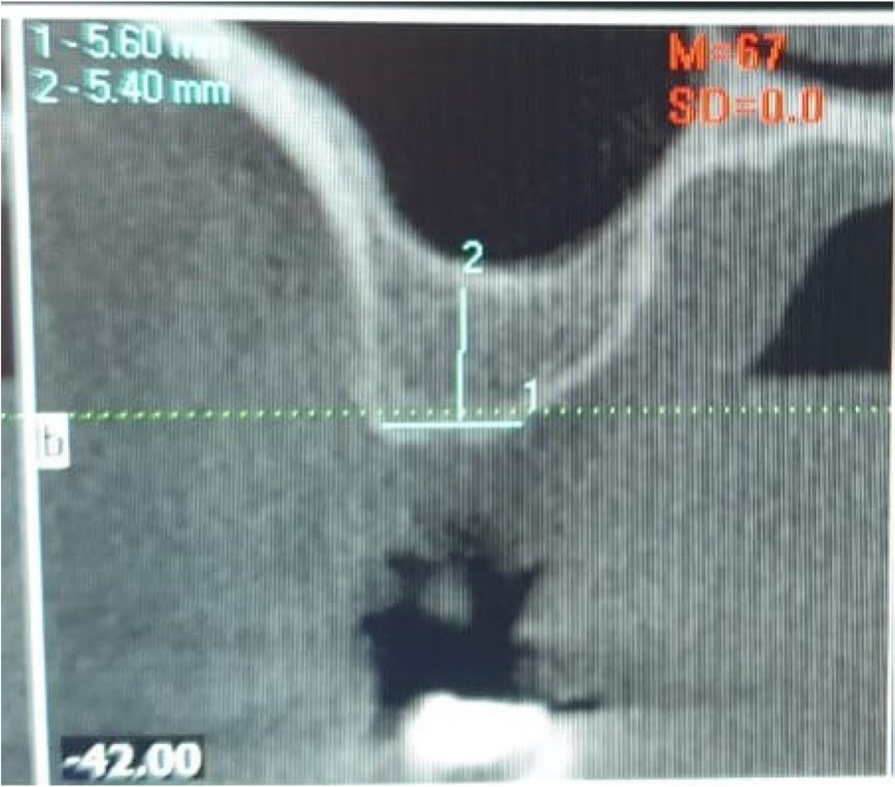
Per-operative CBCT cross-section cut
